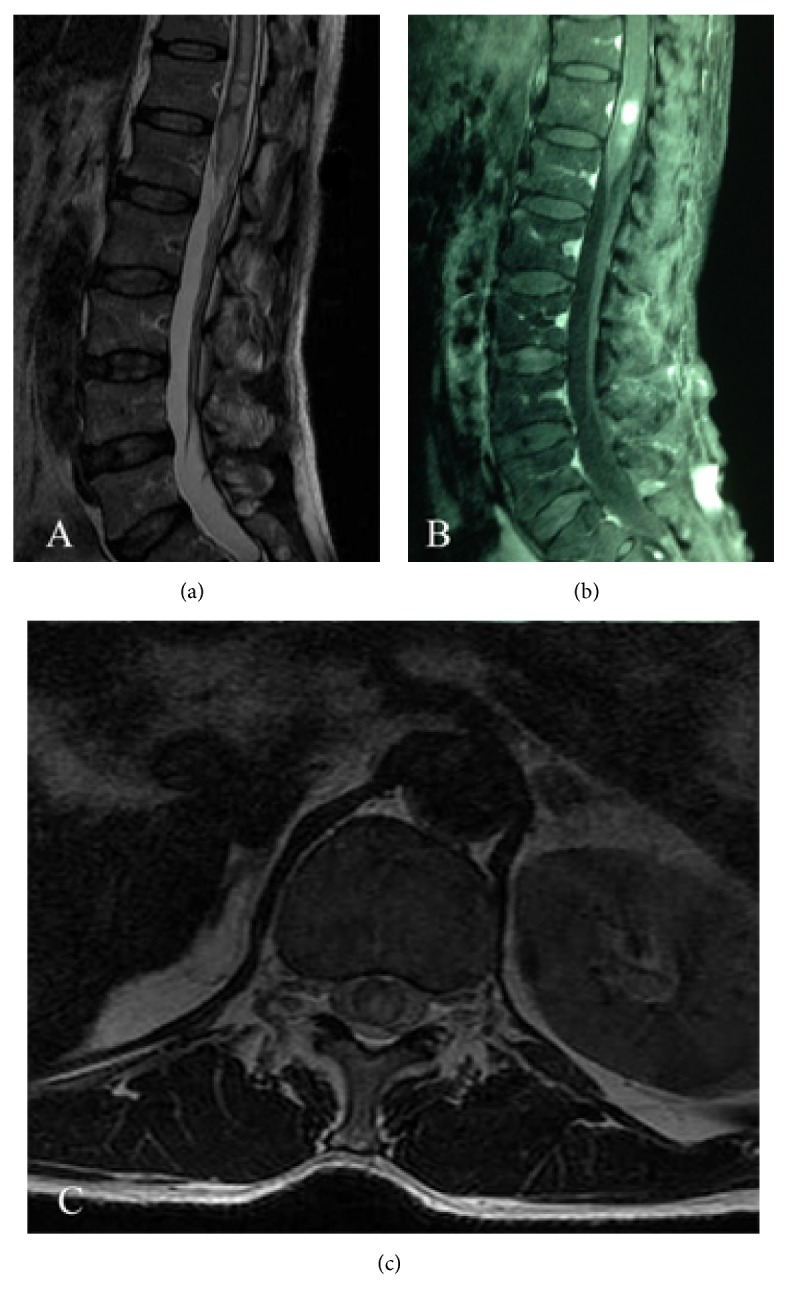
(a) Preoperative T2-weighted sagittal MRI demonstrating increased signal intensity. (b) Preoperative T1-weighted sagittal MRI revealing a well-defined tumor with significant enhancement after gadolinium injection. (c) Preoperative T1-weighted axial MRI showed well-circumscribed lesion inside the spinal cord at the T12 level.
